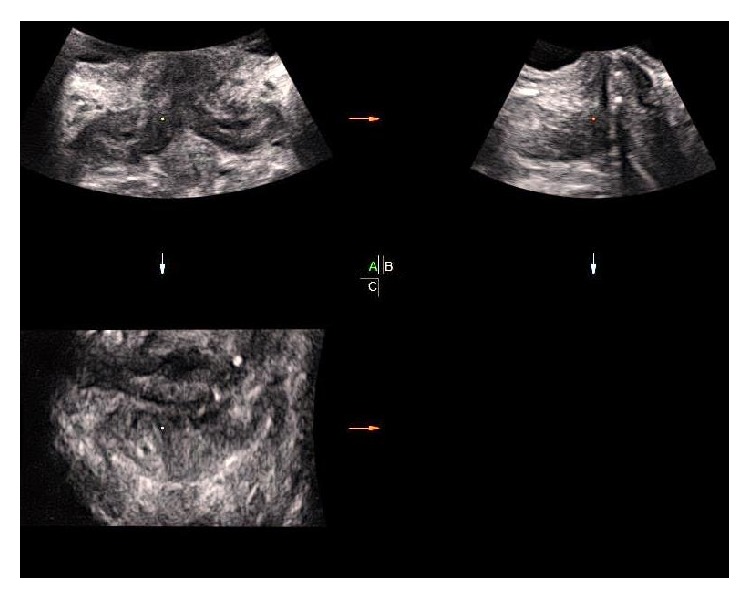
Postoperative 3D ultrasound imaging.
